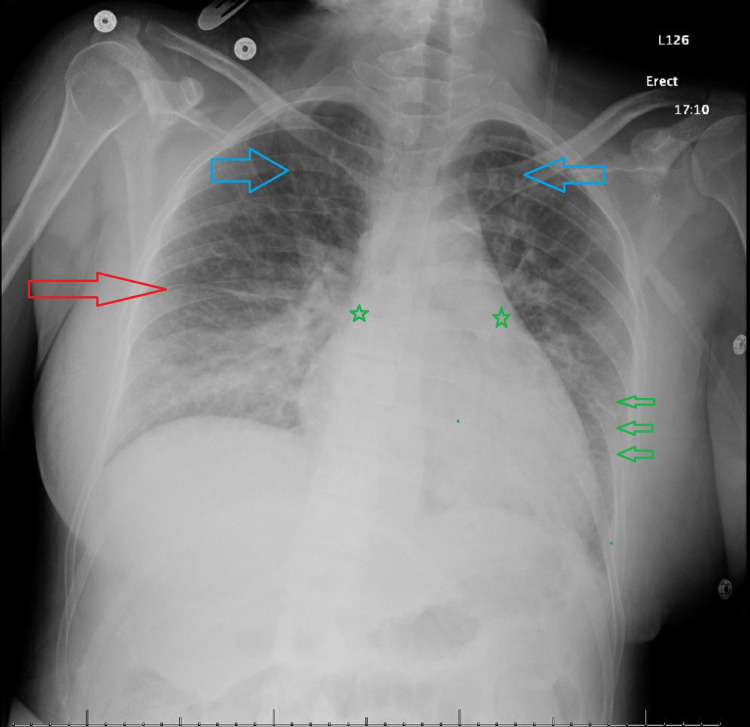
Chest X-ray of our patient showing the picture of the pulmonary edema. Red arrow: fluid in the transverse fissure; green arrows: Kerley B lines; blue arrows: cephalization of blood vessels; green stars: fullness in the hilum
